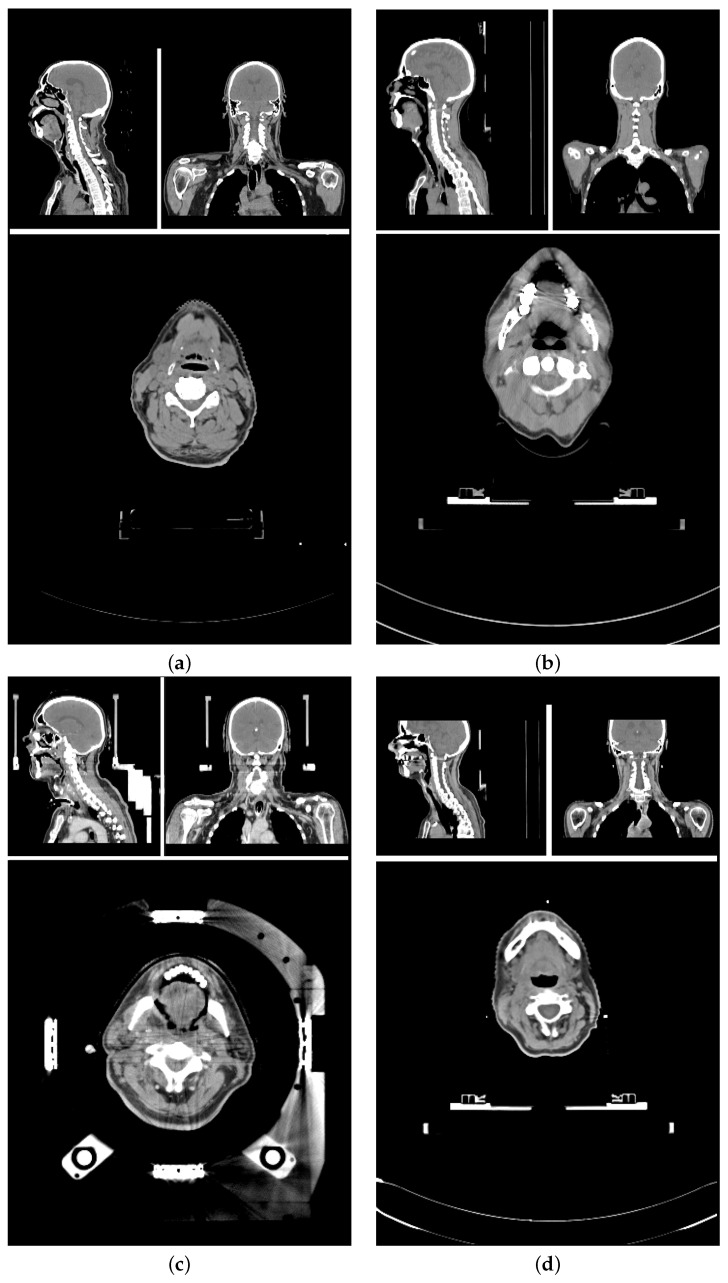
Screenshots of planning CT scans from exemplary patients of all four cohorts in sagittal, coronal and transversal view. (a) Open access HNC data set [40,41,42], (b–d) in-house HNC data sets. All cohorts differ in their scanning set-up using different treatment couches and immobilization devices. (b) Shows artifacts due to dental implants, and (c) shows artifacts due to the stereotactic frames and underwent tracheostomy.
